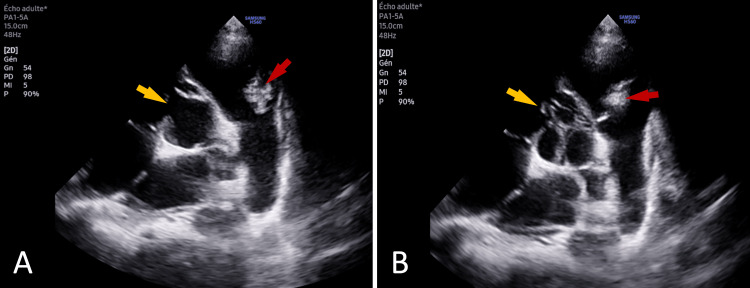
Transthoracic echocardiographic view in the parasternal short axis showing a perimembranous ventricular septal defect (yellow arrows) with a large vegetation on the pulmonary valve (red arrows), during systole (A) and during diastole (B).
